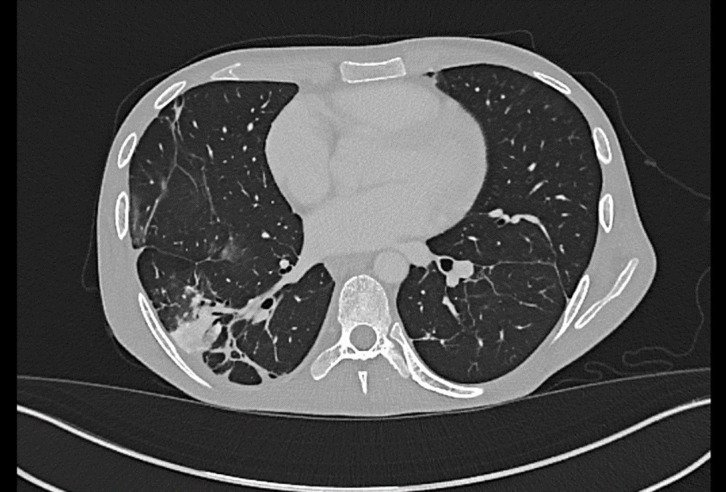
CT scan of the thorax after two cycles of chemotherapy showing regression of metastases.
